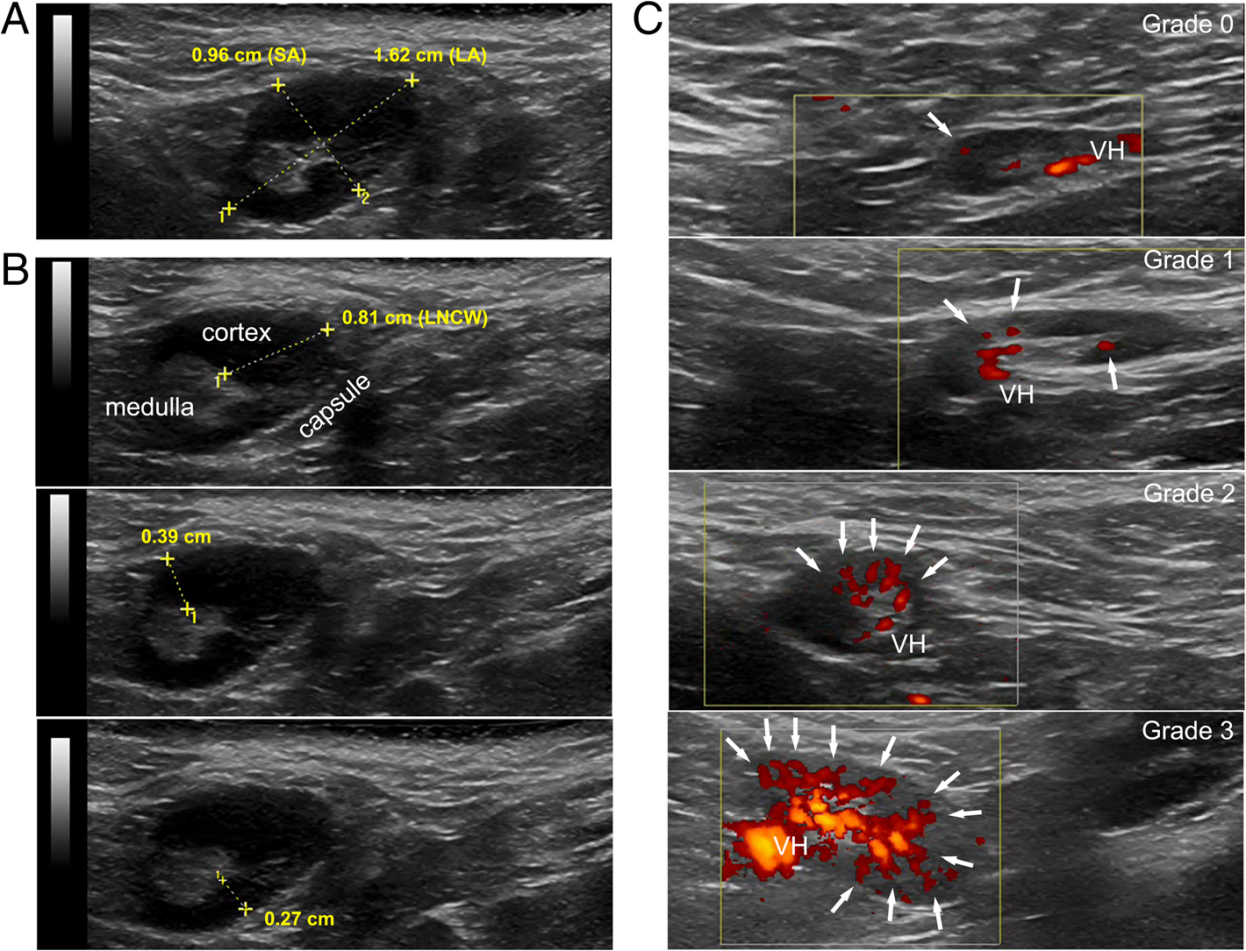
B-mode and power Doppler parameters assessed in axillary LNs by ultrasonography. a Representative image of an axillary LN showing the short axis (*SA*) and long axis (*LA*) used for volume calculation (see Methods for description of the formula). b Representative images of the same axillary LN showing three independent cortical measurements parallel to LN axes (upper, middle, and lower panels). The major of the cortical measurements (presented in the upper panel) is defined as lymph node cortical width (*LNCW*). The capsule, the hypoechoic cortical area (cortex), and the hyperechoic medullary region (medulla) of the LN are outlined. c Representative images of the PD grading system. Four different axillary LNs characterized by progressive PD grades with progressive involvement of the cortical region are shown. *White arrows* indicate the number of PD-positive signals in the cortex. *VH* vascular hilum (anatomic entry site of blood vessels into the node)
